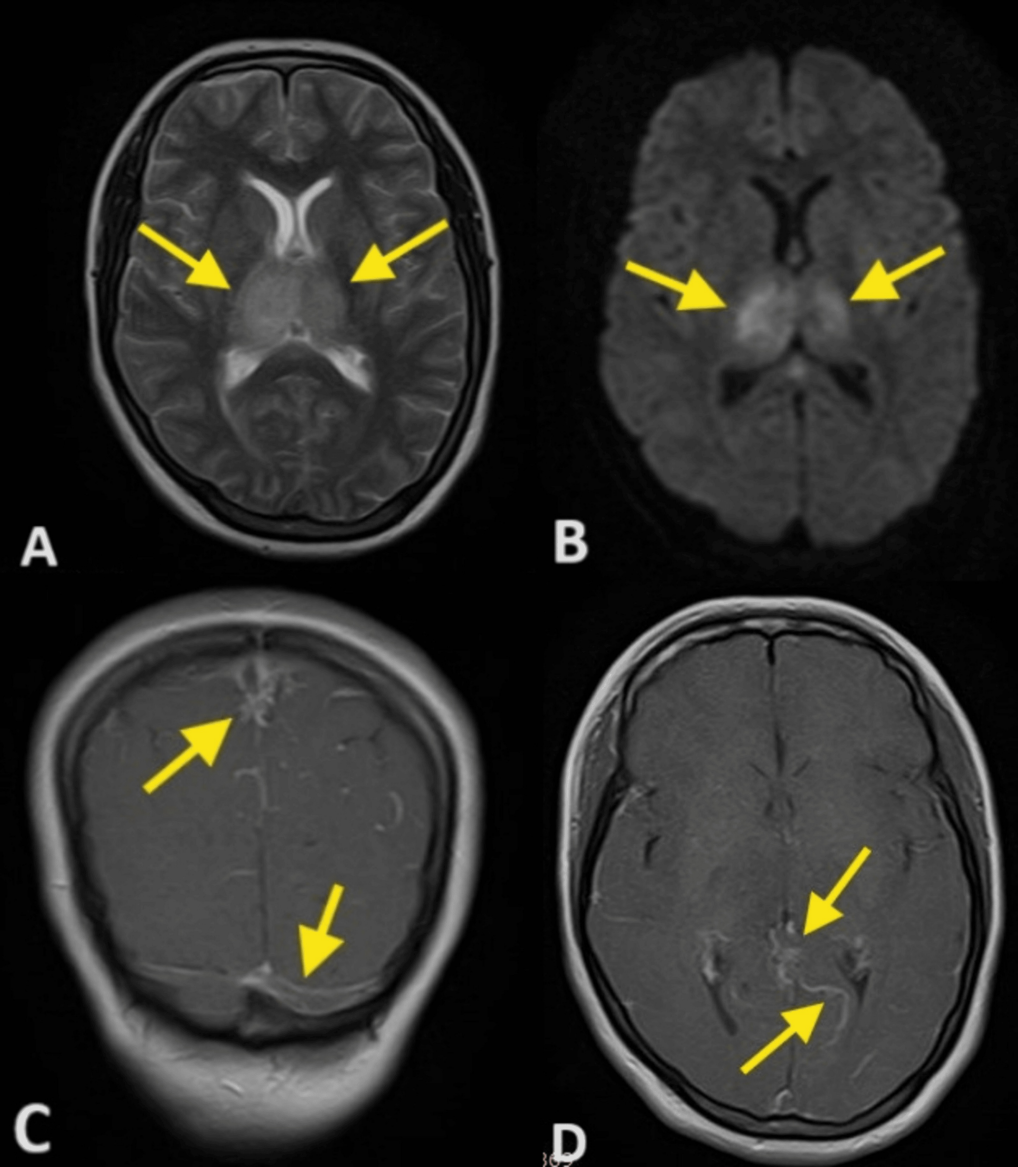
MRI and MRV brain with contrast A: T2-weighted image showing bilateral thalamic hyperintense signals more on the right side (yellow arrows); B: Diffusion-weighted image demonstrating diffusion restriction in bilateral thalamic regions suggestive of thalamic infarcts (yellow arrows); C&D: Post-contrast images showing leptomeningeal enhancement and filling defects in the sagittal and transverse sinus (yellow arrows) MRI: magnetic resonance imaging; MRV: magnetic resonance venography
